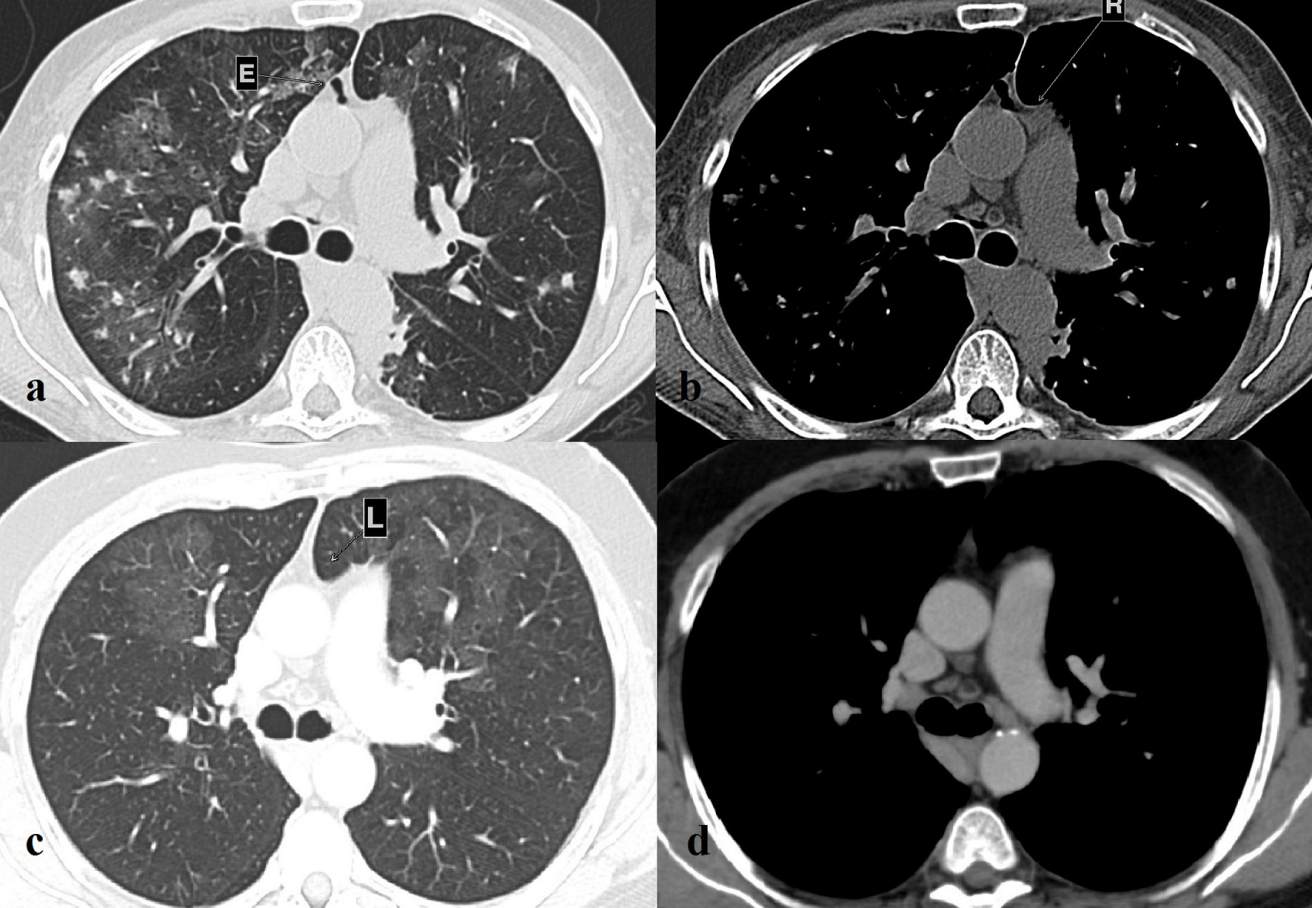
(a). Lung window: a moderate mediastinal emphysema (E) is evident. (b). Mediastinal window: the axial image shows the pre-aortic recess (R) slightly filled with fluid. In addition, the region between the ascending aorta and main pulmonary artery shows a blunt morphology. (c). Lung window: CT performed 2 years later shows interval changes of the region between the ascending aorta and main pulmonary artery. A small amount of lung interposition between the aorta and pulmonary artery (L) is evident, mimicking a condition of CAP. (d). Mediastinal window: the aortopulmonary junction shows a sharp morphology. CAP, congenital absence of pericardium.
